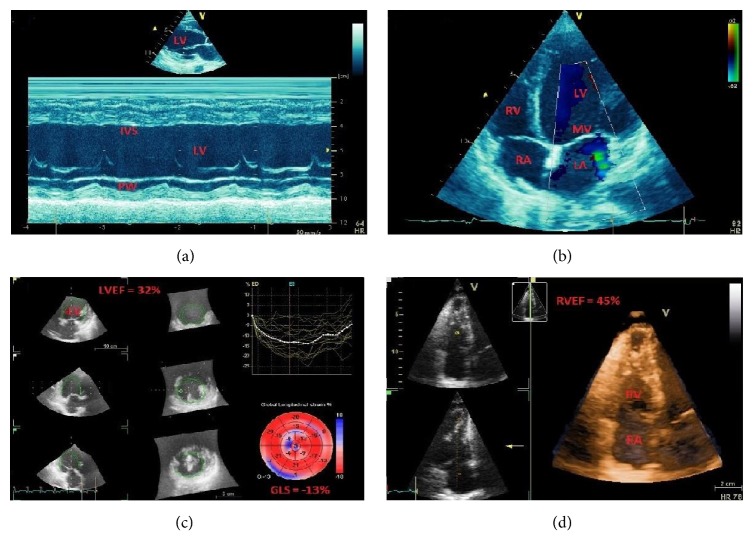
A 48-year-old male patient with dilated cardiomyopathy from previous myocarditis and systolic dysfunction. Patient was on medical therapy. (a) LV dilatation and septal (IVS) and posterior wall (PW) hypokinesia by M-mode echocardiography. (b) Two-dimensional four-chamber apical view showing LV end-systolic dilatation and mitral valve (MV) regurgitation. (c) Three-dimensional four-chamber apical view showing LV dilatation and severe systolic dysfunction (left ventricular ejection fraction = 32%). (d) Calculation of three-dimensional right ventricular volumes showing right ventricular ejection fraction at lower normal limits (45%). GLS = global longitudinal strain; IVS = interventricular septum; LV = left ventricle; LVEF = left ventricular ejection fraction; PW = LV posterior wall; RA = right atrium; RV = right ventricle; RVEF = right ventricular ejection fraction.
